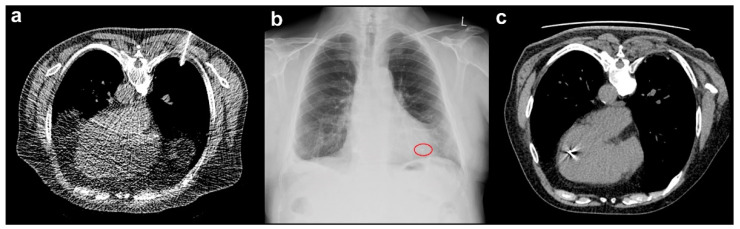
A representative case of marker migration. (a) Computed tomography (CT)-guided fiducial marker implantation is used to place a marker into a metastasis in the lower lobe of the right lung. (b) Radiography control with the projection of the marker to the heart. (c) The marker is shown after migrating to the left ventricle on the day of insertion. There has been no change in the marker position after two years.
